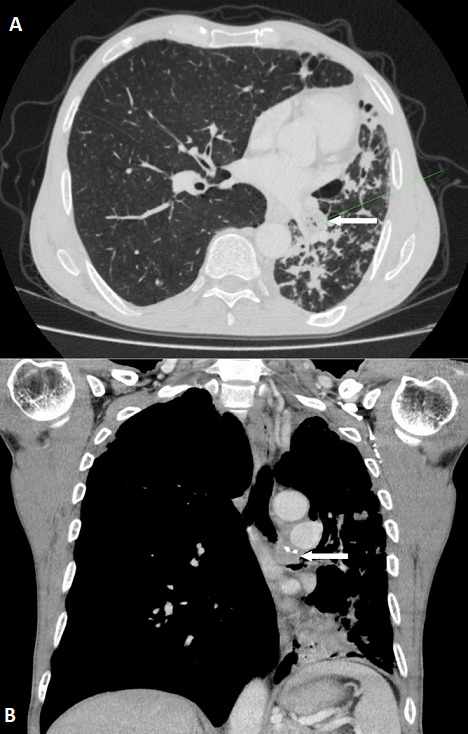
Chest CT scan-case 1: (A) axial CT scan image; (B) coronal CT scan image. Left-sided bronchial-centric mass in the left main bronchus (white arrow) associated with multiple left hilar lymph nodes and carcinomatous lymphangitis in the left inferior lobe
